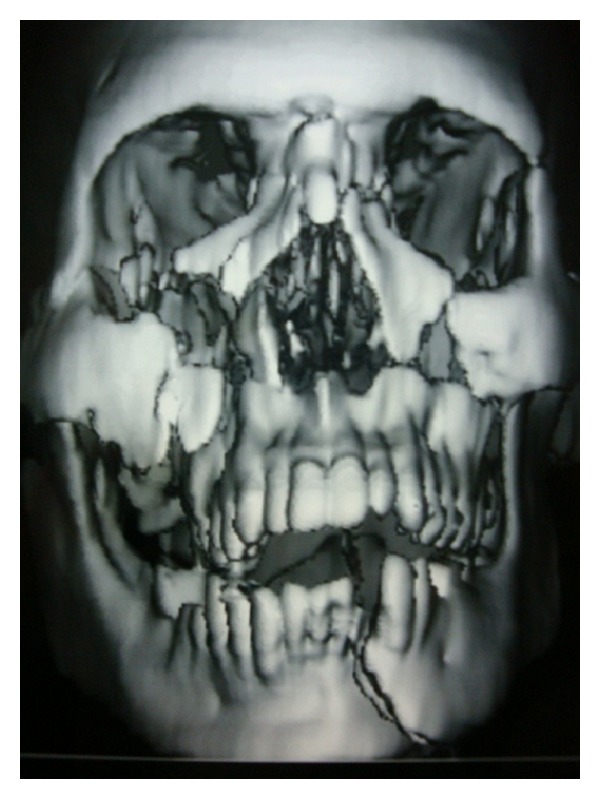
Three-dimensional computed tomography scan showing multiple fractures of jaw bone.
